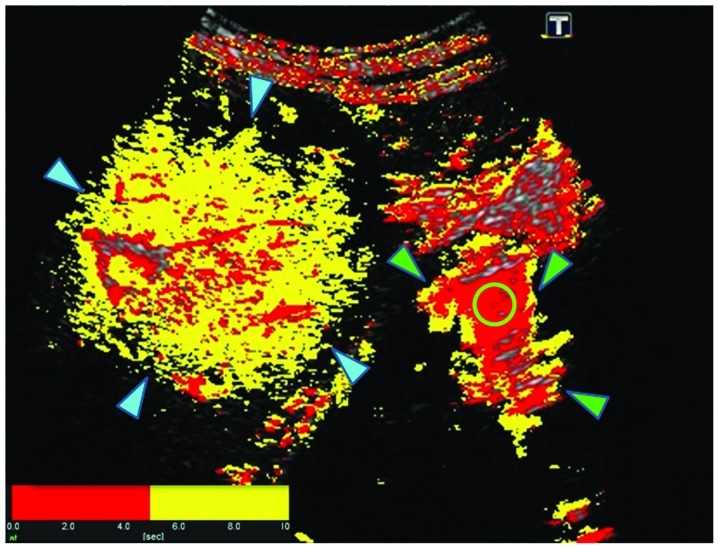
Sonazoid-enhanced ultrasonography (US) image with arrival time parametric imaging (At-PI) taken during an episode of jaundice (hospital day 9; Arrow heads: Blue indicate the liver and green indicate the kidney). The majority of the liver parenchyma was yellow, with a ratio of red (ROR) of 15.7%.
